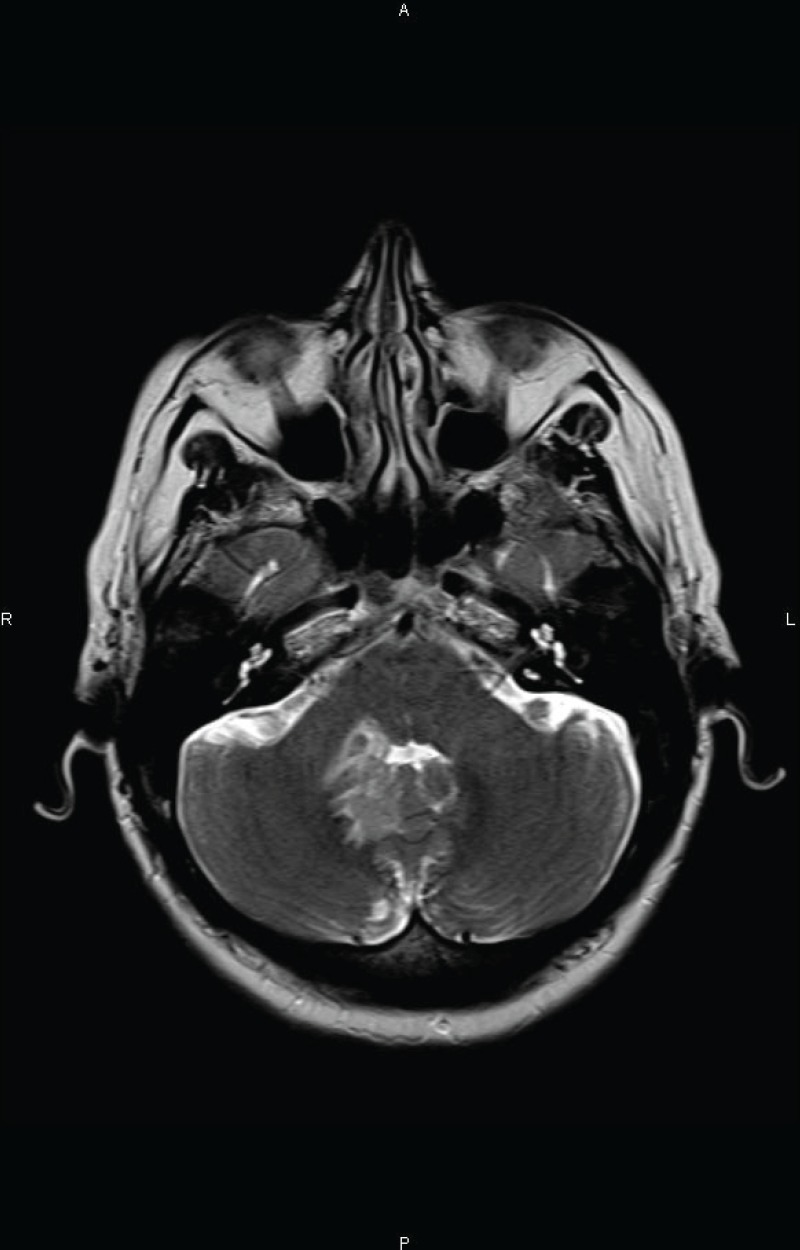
Axial T2 weighted MR image at the level of the posterior fossa revealing a slightly hyperintense infiltrating lesion adjacent to the right side of the fourth ventricle.
